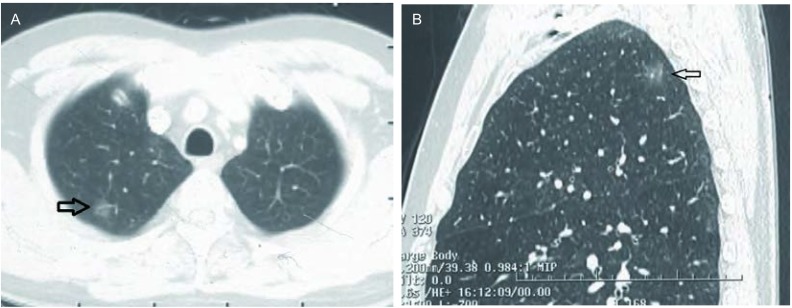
胸部CT检查。A：肺窗水平面显示右肺上叶尖端磨玻璃样结节（箭头所指）；B：矢状面显示右肺上叶尖端磨玻璃样结节（箭头所指）。 CT scan of chest. A: In lung window, horizontal, show the ground-glass nodule in apical segment of the superior lobe of the right lung (arrowhead); B: Sagittal, show the ground-glass nodule in apical segment of the superior lobe of the right lung (arrowhead).
